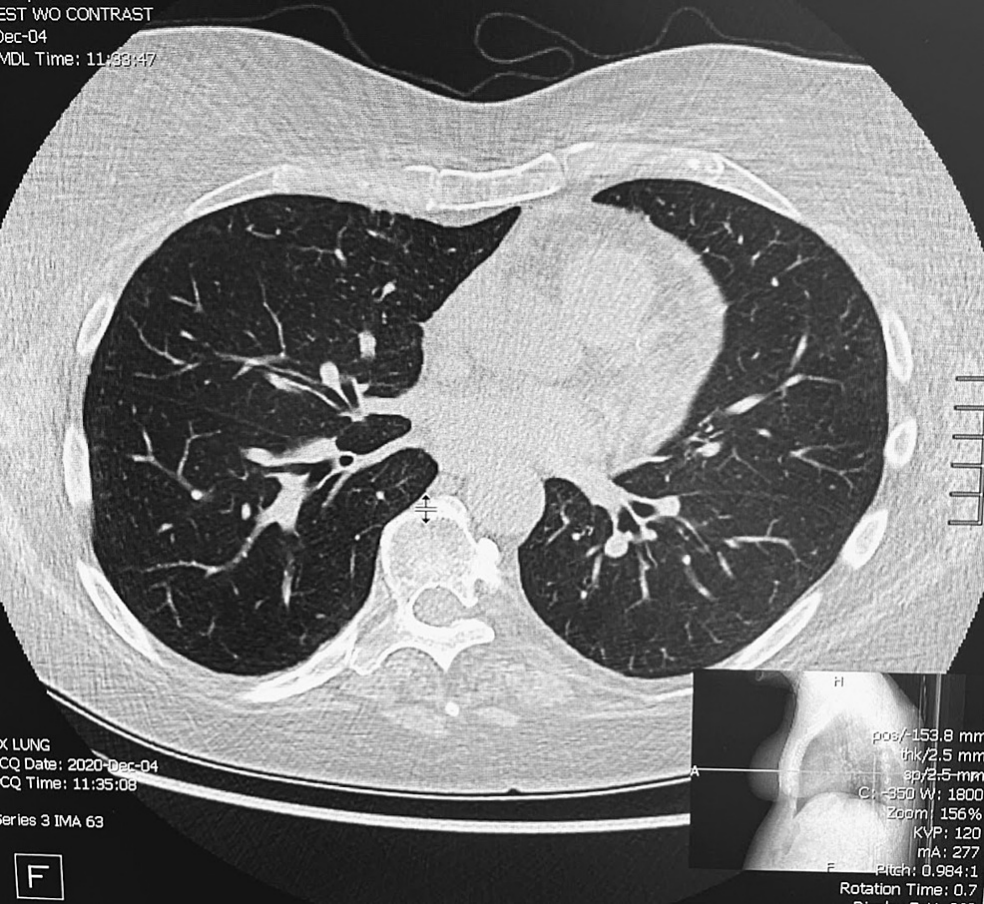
CT image of pulmonary post-infectious changes. The presence of lymphadenopathy and calcified nodules is also noted CT: computed tomography
